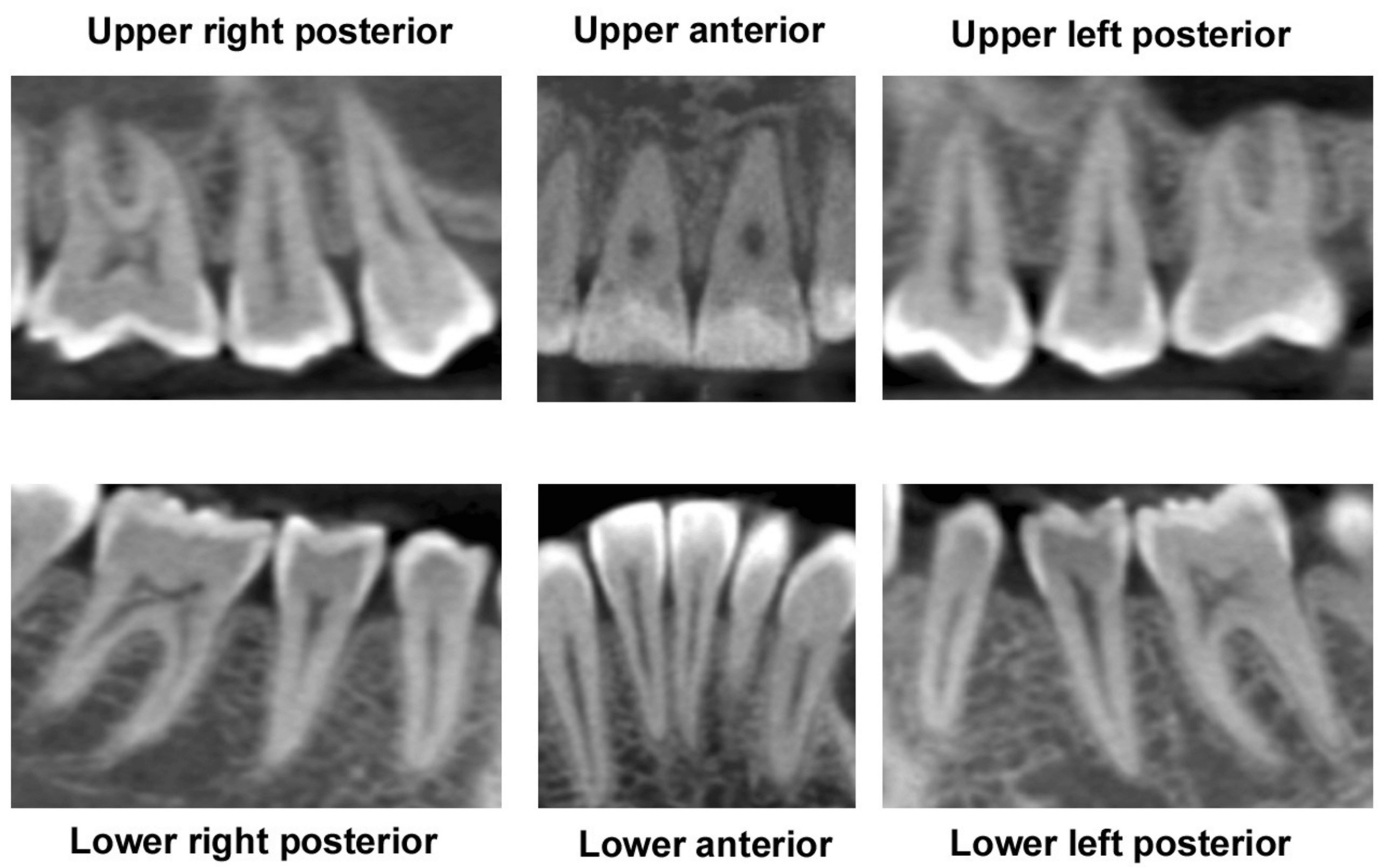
Six radiographic images from a pre-treatment cone-beam computed tomographic (CBCT) scan of the patient. Original CBCT images of a patient from the study.
